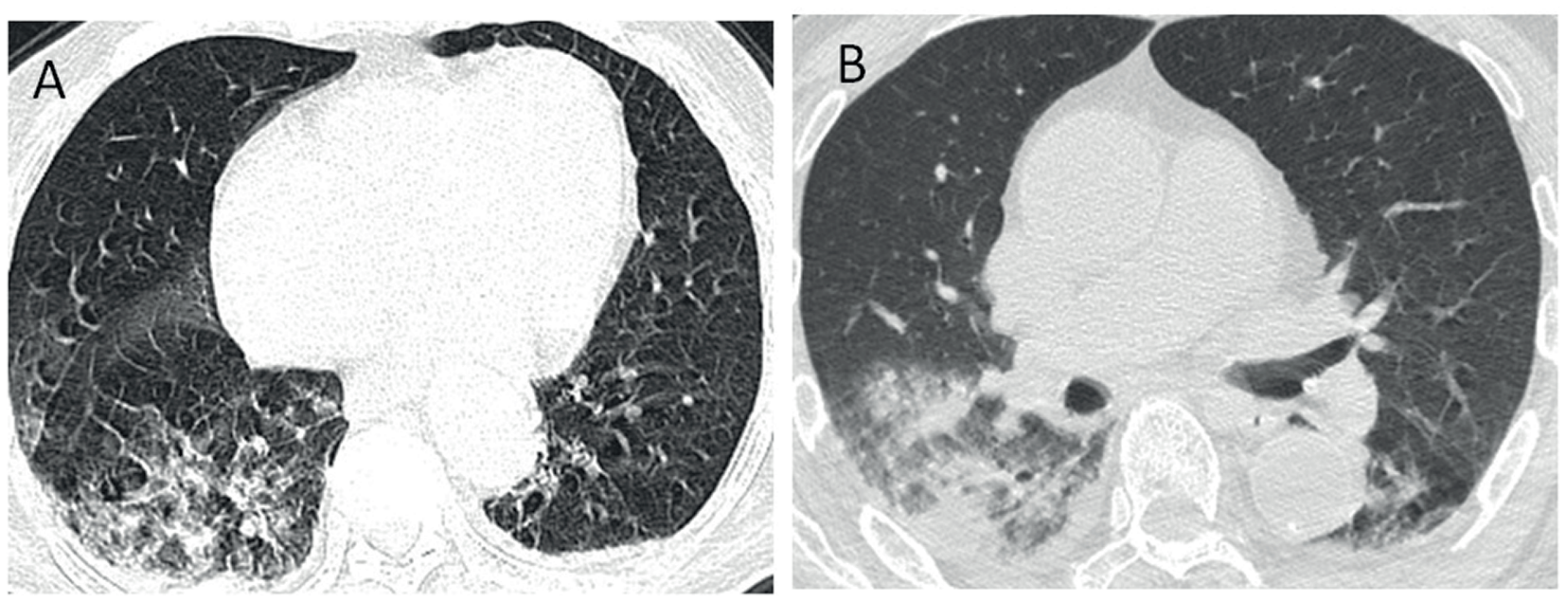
Chest CT images of a COVID-19 patient with PD. Axis chest CT scan showed bilateral multiple ground-glass opacities (A) on admission and consolidation of the right lung and pleural effusion (B) progressed within 48 h, consistent with typical COVID-19 infection. PD, Parkinson’s disease.
